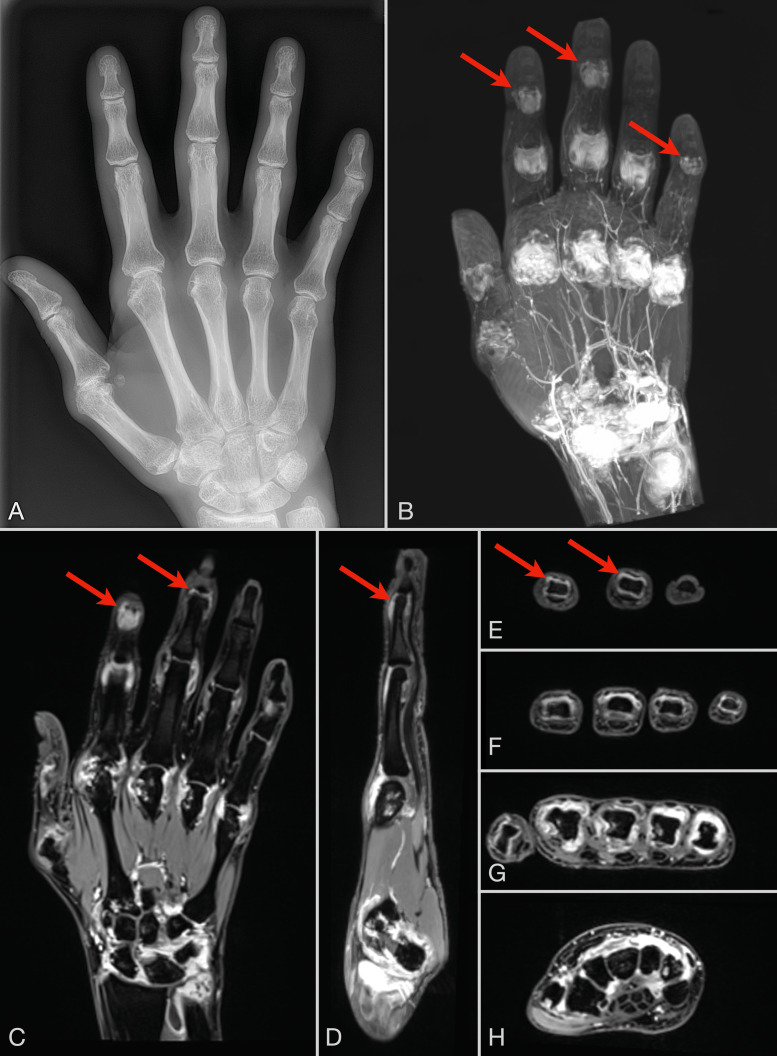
Image of the patient’s right hand with conventional X-ray diagnostics (A) and MRI with contrast agent gadobutrol (B-H). The maximum intensity projection (B) clearly shows all joints affected by synovial inflammation, including involvement of the distal interphalangeal (DIP) joints (red arrows). The individual sections in coronal (C), sagittal (D, 3rd finger), and transverse (E, DIP joints; F, proximal interphalangeal joints; G, metacarpophalangeal joints; H, intercarpal joints) slices confirm the synovial involvement of all joint levels. The red arrows mark the DIP joints.
